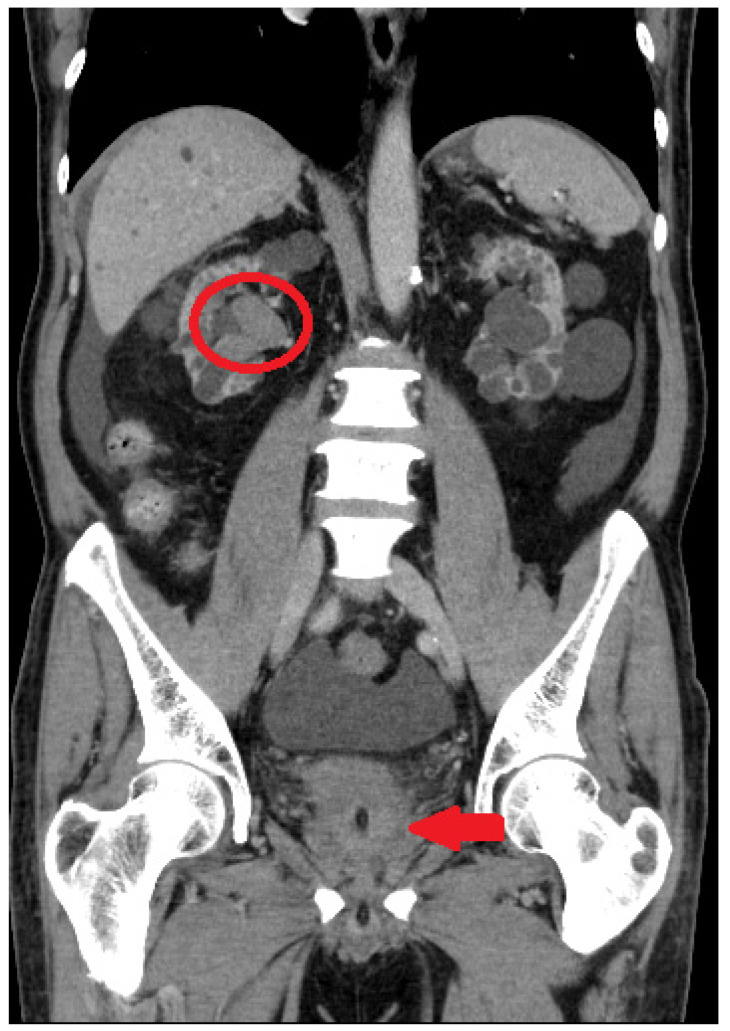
Initial assessment with computed tomography. Dense blood clot (red arrow) and right hydronephrosis (red circle) were observed.
